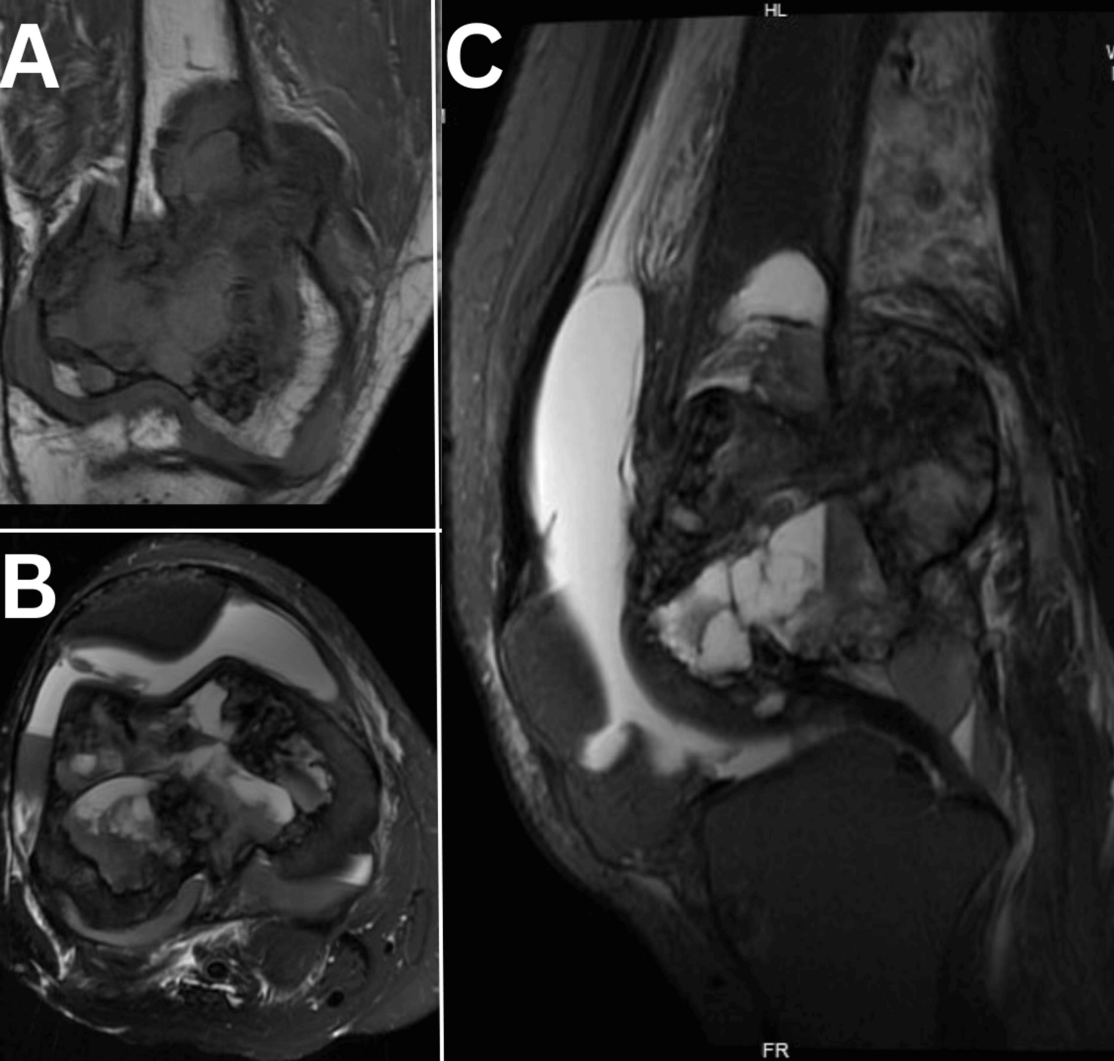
MRI of the right knee demonstrating (A) the coronal T1-weighted image (T1WI), (B) axial fat-suppressed (FS) T2-weighted image (T2WI), and (C) sagittal FS T2WI. These images reveal a well-defined, multilobulated, expansile lesion in the subarticular region of the distal femoral condyle. The lesion appears iso- to hypointense on T1WI and iso- to hyperintense on FS T2WI, with the presence of fluid-fluid levels. Inferiorly, it extends to the joint margin of the knee, while laterally, it causes cortical breach, leading to adjacent soft tissue involvement and edema.
